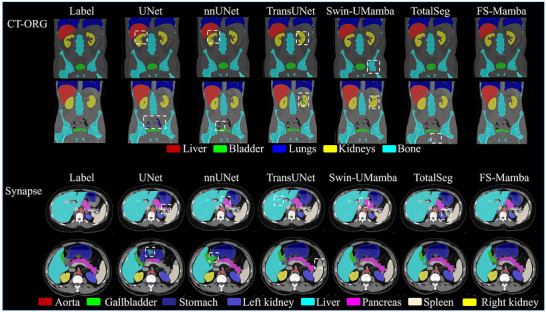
Comparison results of FS‐Mamba with other models on CT‐ORG and Synaspe datasets, with white boxes marking several regions that differ significantly from the reference image. FS‐Mamba has fewer segmentation errors on organs and tissues.
